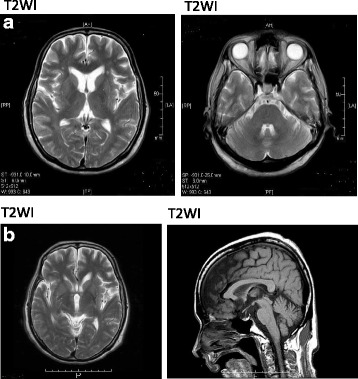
Brain magnetic resonance images of the patient at 58 years of age (spring 2015) (a) and 59 years of age (winter 2015) (b). a Putaminal atrophic change and low- and high-intensity signals of the lateral rim of the putamen were observed predominantly in the left putamen in the T2-weighted image (T2WI). However, T2WI did not show the hot-cross bun sign, which may reflect pontine atrophy. b As in the previous year, magnetic resonance imaging findings revealed putaminal atrophic change and both low- and high-intensity signals of the lateral rim of the bilateral putamen, again predominantly in the left putamen. There were slight atrophic changes in the cerebellum and brain stem
